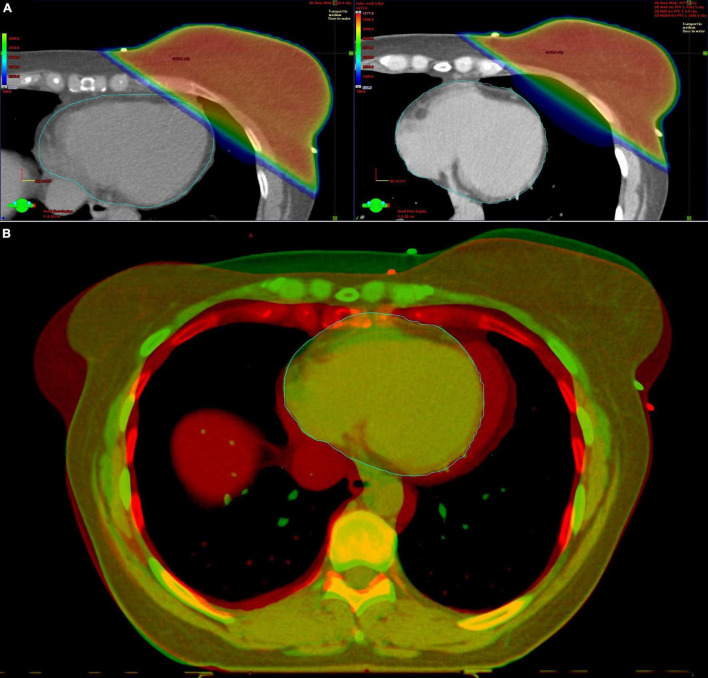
Deep inspiration breath hold (DIBH) radiotherapy for left-sided breast cancer. (A) Axial computed tomography slices with radiotherapy plan at the same level of the breast in free breathing (left) and DIBH (right). The distance between the heart and the breast is greater in DIBH, minimizing the radiation of the heart. (B) Fusion image of the free breathing (red color) and DIBH (green color) computed tomography scan.
